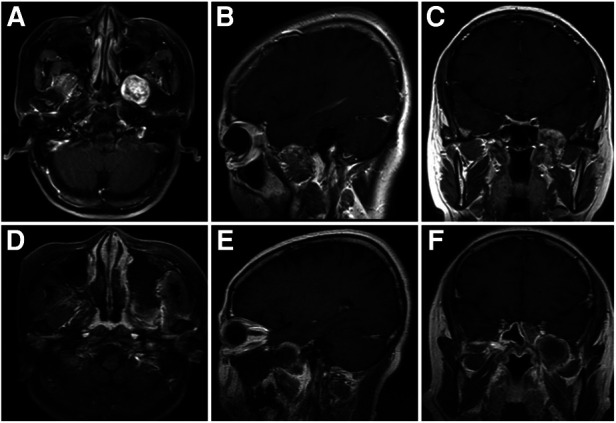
Case 2: a 34-year-old man with a schwannoma in the ITF. A preoperative T1-weighted enhanced MRI (A–C) shows the tumor located in the ITF. A postoperative T1-weighted enhanced MRI confirms the total removal of the tumor by the PRLMA (D–F). The lateral wall of the nasal cavity remains intact (D).
